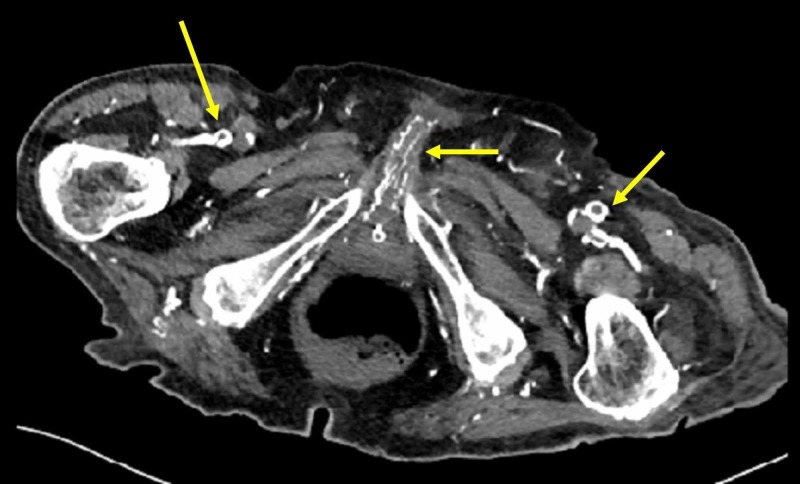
Axial view of the abdominal/pelvic CT scan The image shows diffuse calcifications of the femoral arteries and their branches of division and penile arteries tributary to the internal pudendal artery (yellow arrows) CT: computed tomography
